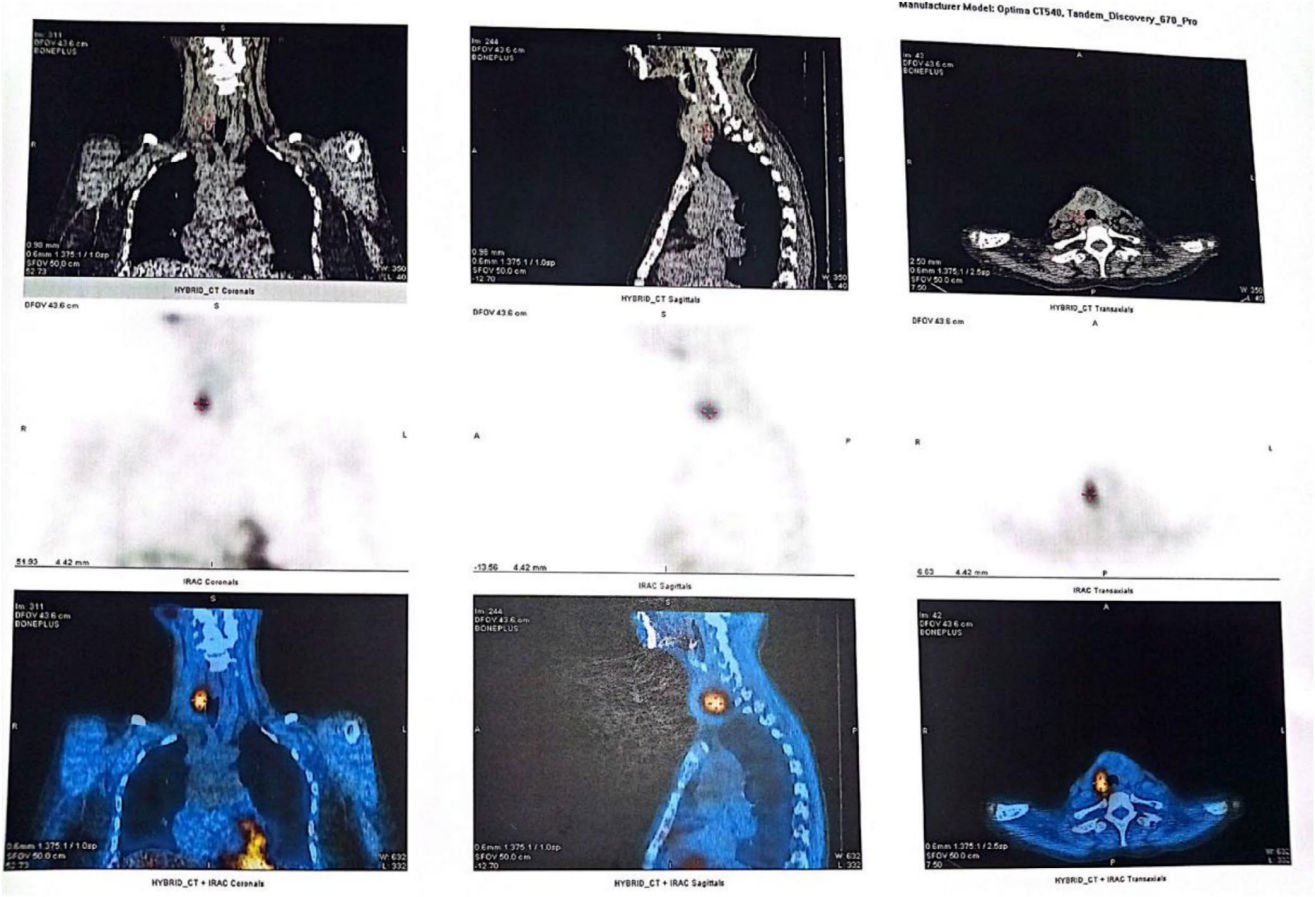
SPECT CT images showing a focal area of increased tracer uptake posterior to the enlarged right thyroid lobe. The thyroid gland appears enlarged and has a nodular appearance.
